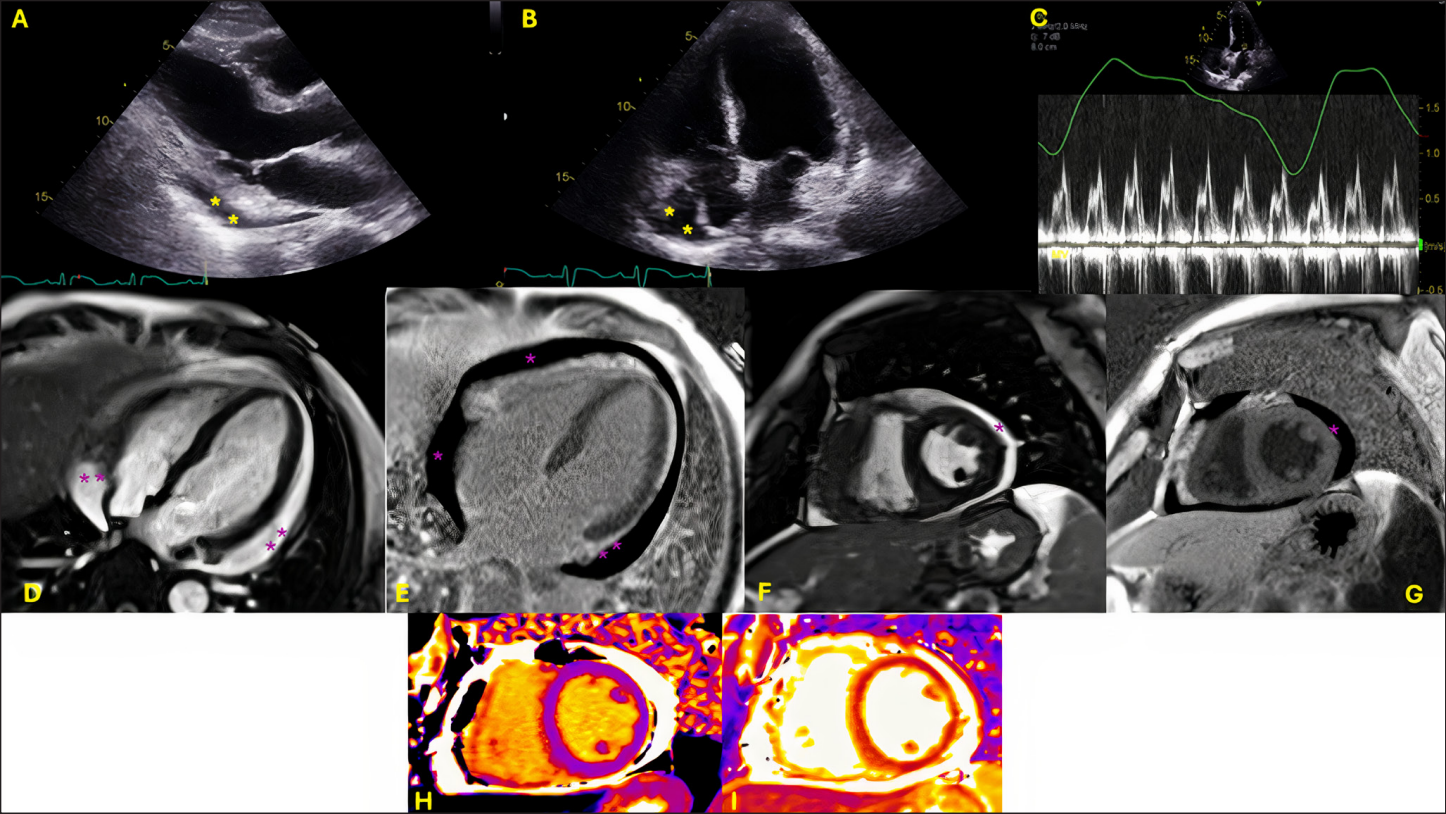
(A) Transthoracic echocardiogram, parasternal long-axis image with small posterior pericardial effusion (asterisk), (B) transthoracic echocardiogram, apical 4-chamber view with anterior pericardial effusion (asterisk). (C) Pulsed wave Doppler across the mitral inflow showing respiratory variation > 25% consistent with increased intrapericardial pressures. (D, F) Cardiovascular magnetic resonance imaging, balanced steady-state free precession sequence in apical 4-chamber (D) and short-axis view (F) showing circumferential pericardial effusion (moderate, asterisk). (E, G) Late gadolinium enhancement imaging suggests nulled myocardium with no evidence of ischemic damage or myocarditis. (H, I) T1 and T2 parametric mapping.
